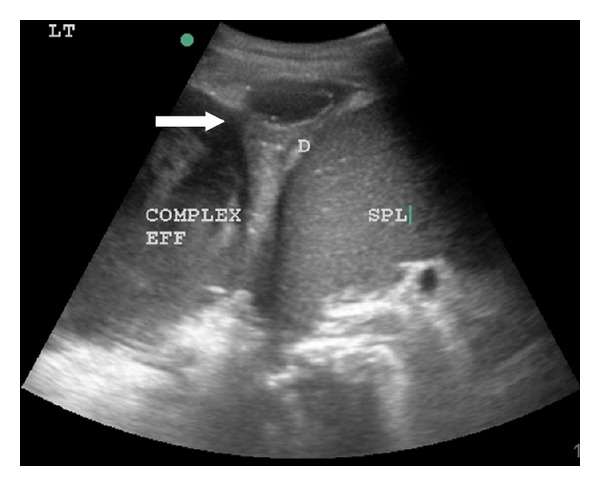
Ultrasound appearance of complex pleural effusion with extension through diaphragm (white arrow). D: diaphragm. SPL: spleen.
